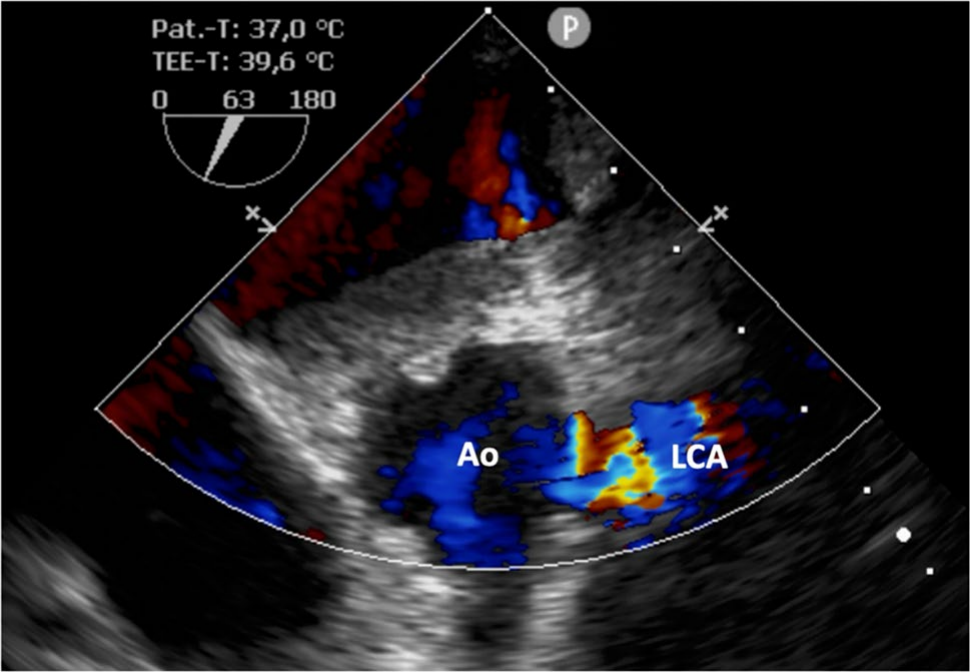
TEE imaging after connection of the LCA with the aortic root. Figure 4 shows blood flow from the aorta through the reinserted LCA. *Ao* aorta, *LCA* left coronary artery, *TEE* transesophageal imaging
